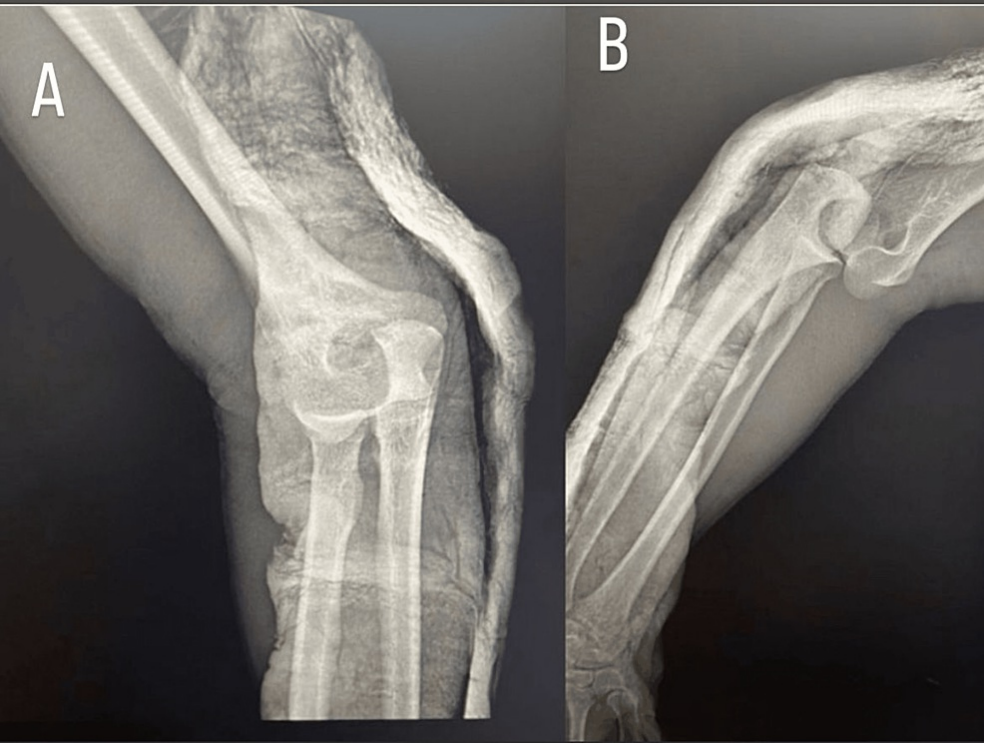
Radiographic view after closed reduction, where joint congruence is restored. (A) Anteroposterior (AP) view of elbow X-ray. (B) Lateral view of elbow X-ray
